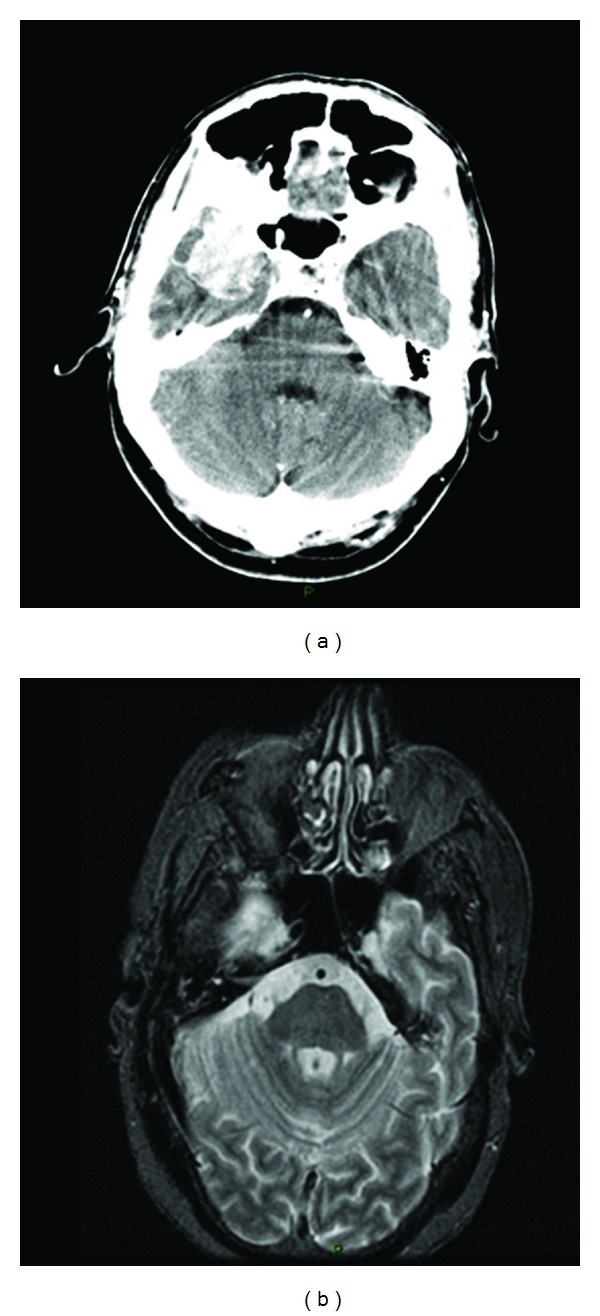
CT scan (a) and MRI (b) revealed a 4.5 × 4.5 × 3-cm mass that enhanced with contrast, in the right greater wing of the sphenoid bone which was invading the anterior pole of the temporal lobe and the sphenoid sinus.
